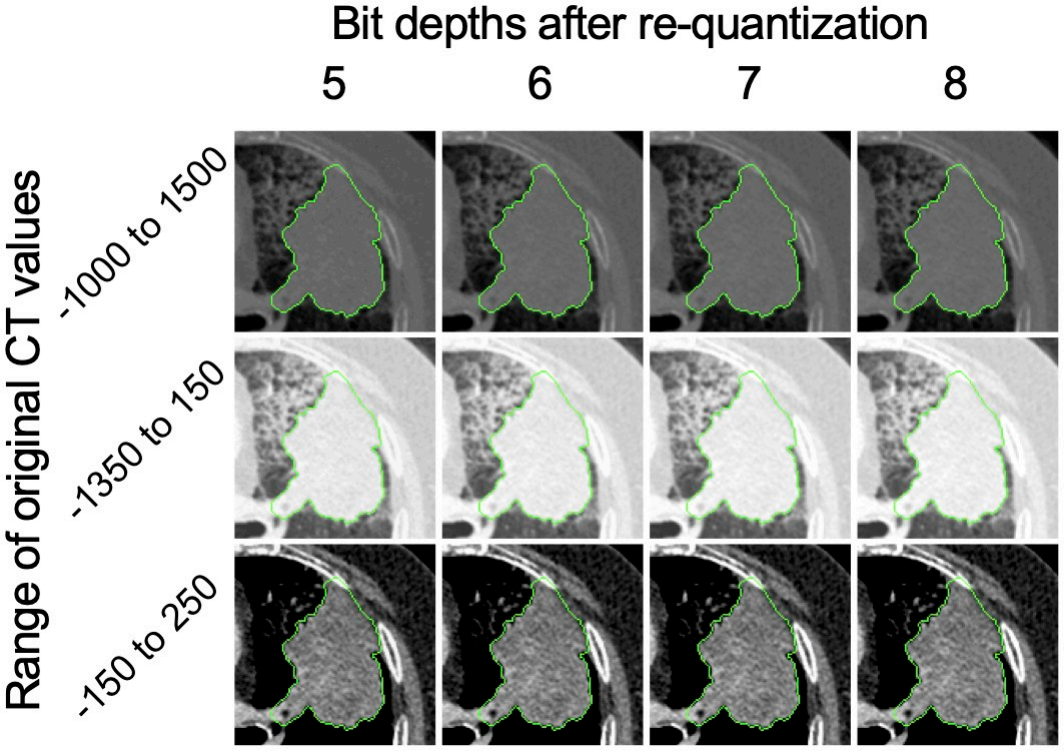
q*-bit images generated from the computed tomography (CT) images using four ranges of Hounsfield units (HU) of CT images (-1000 to 1500 HU, -1350 to 150 HU [lung range window], and -150 to 250 HU [mediastinal range window]) with four bit-depths after re-quantization (5, 6, 7, and 8 bits).
